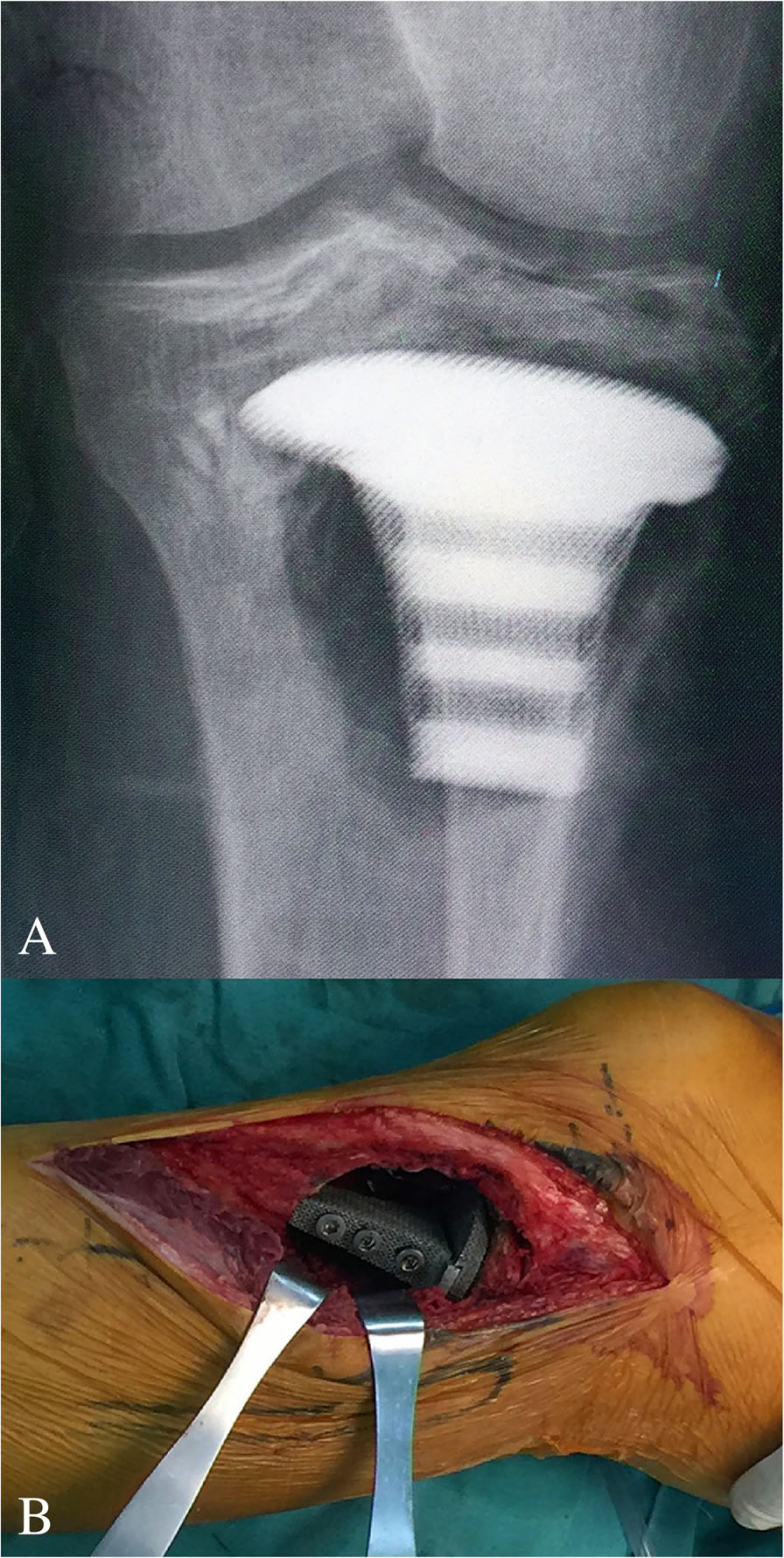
a-b. Intraoperative pictures: (a) confirming the location of implant by the X-ray; (b) indicating screws fixation after the confirmation of implant position
